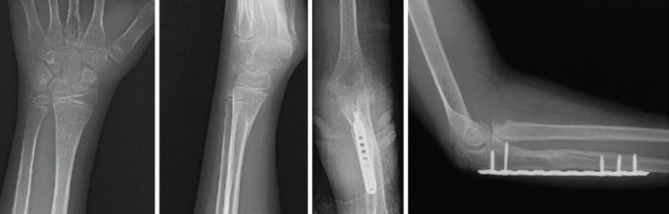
Six-month post-operative radiographs showing complete healing of the ulnar and radial fractures with maintained alignment and hardware in situ. Full restoration of the anatomical relationship between the ulna, radial head, and distal radius was noted.
